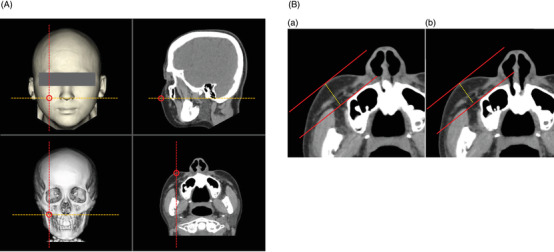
Measurements of the cheek thickness. (A) Reconstructed images on a workstation. On a workstation, the thickest point of the cheek, known as the malar top, was accurately determined using reconstructed sagittal and axial images. The malar top was double‐checked through observations from the anterior side of the face by using a three‐dimensional computed tomography (3DCT) image. On 3DCT images of the face and facial bone, a sagittal image is shown as a red dotted line, and an axial image is shown as an orange dotted line. Spiral CT imaging data have a coordinate axis in the imaging space. Thus, the same point can be precisely indicated on 3DCT images of the face and facial bone, as well as all multiplanar reformatted axial and sagittal images by utilizing the spiral CT coordinates. The centers of the red open circles indicate shared coordinates. (B) Measurements of the cheek thickness both pre‐ and post‐ facial massage. (a) A reconstructed axial image pre‐massage. First, a straight red line was drawn along the surface of the facial bone on a reconstructed axial image of the pre‐facial massage cheek. Next, a red line parallel to it was drawn tangent to the surface of the malar top. The distance from the facial bone to the surface of the malar top was defined as the cheek thickness of the pre‐facial massage. (b) Reconstructed axial image post‐massage. Finally, a reconstructed axial image of the post‐massage cheek at the same level as that used for the pre‐massage evaluation was created using the stationary facial bone as the basis for measurements, and the post‐massage cheek thickness was measured using the same method as that used for the pre‐facial massage measurement. Change rate of the cheek thickness (%)  = (cheek thickness post‐massage/cheek thickness pre‐massage) × 100
